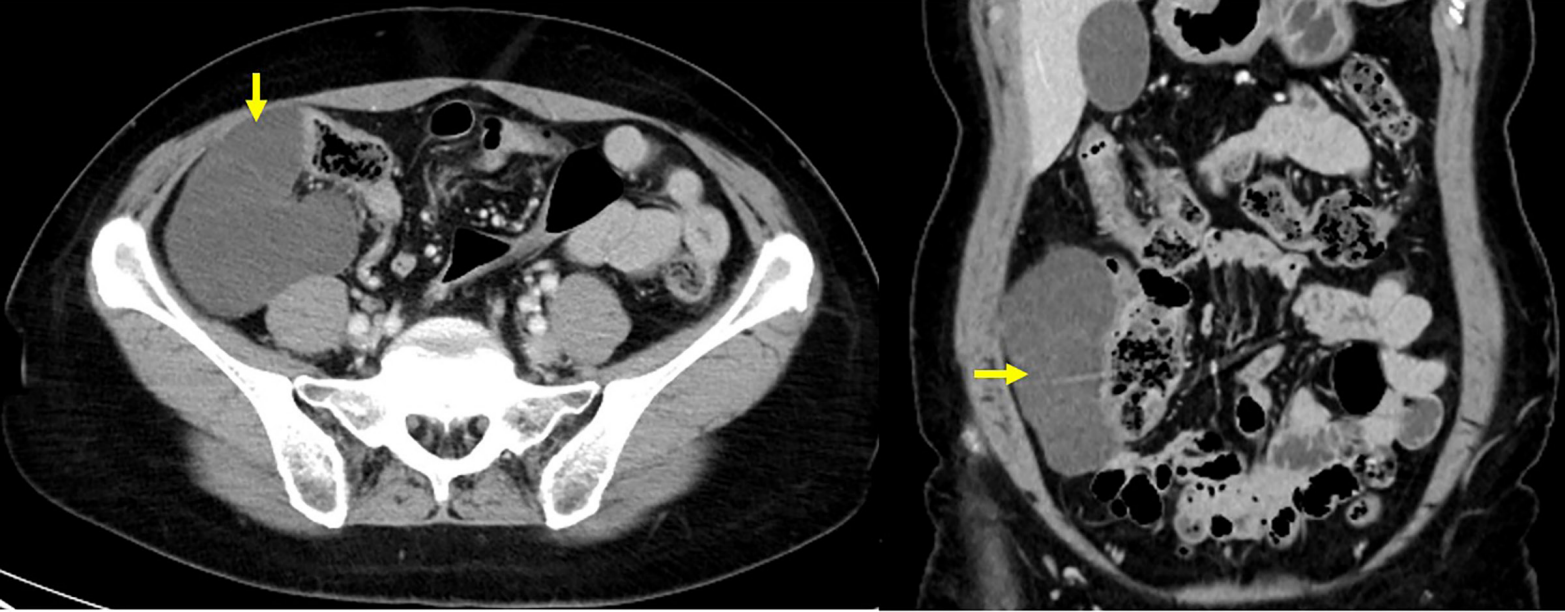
Contrast-enhanced CT findings. The CT images show multicystic lesions located adjacent to the ascending colon. The yellow arrow indicates the tumor.
